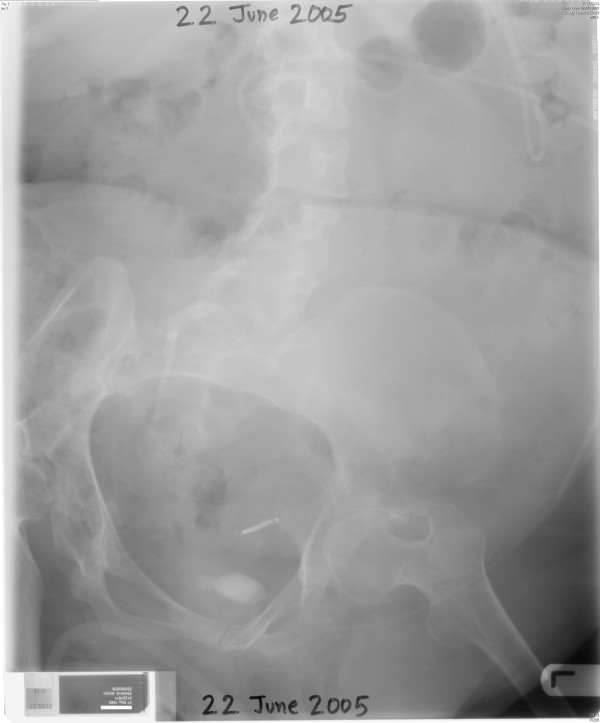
X-ray of abdomen, taken on 22 June 2005, showed vesical calculi.
